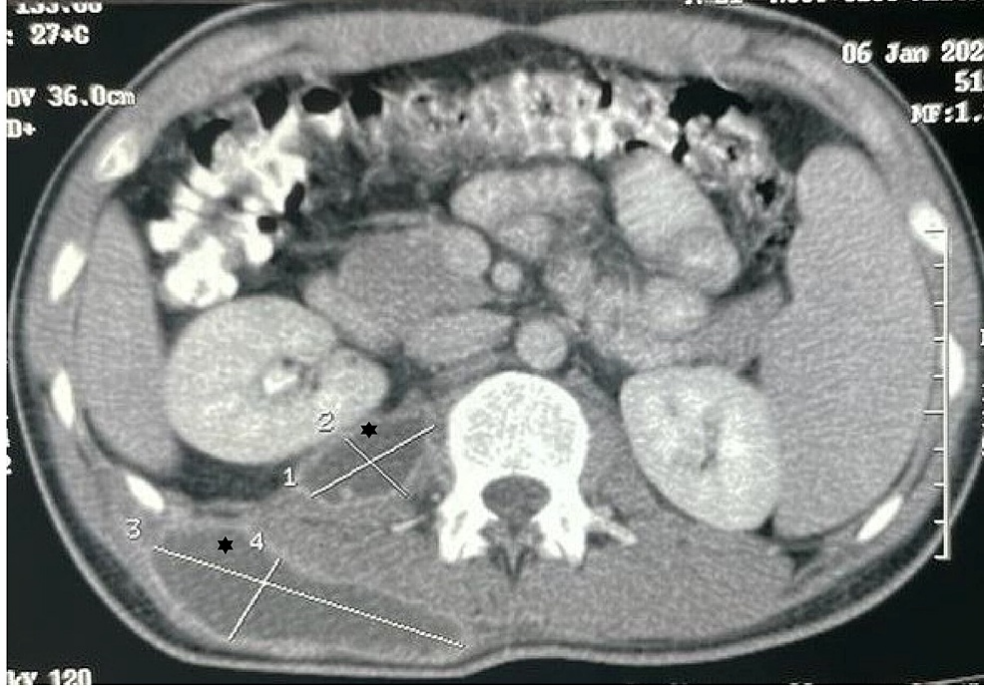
Contrast-enhanced computed tomography (CECT) abdomen showing abscess in psoas major and quadratus lumborum muscles.
